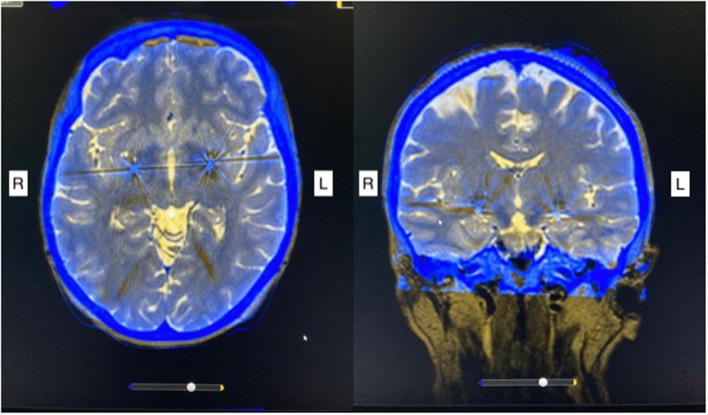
Post-operative stereotactic CT imaging fused with preoperative MRI scan shows bilateral electrode placement in the posteroventral lateral globus pallidus internus. R, right; L, left.
